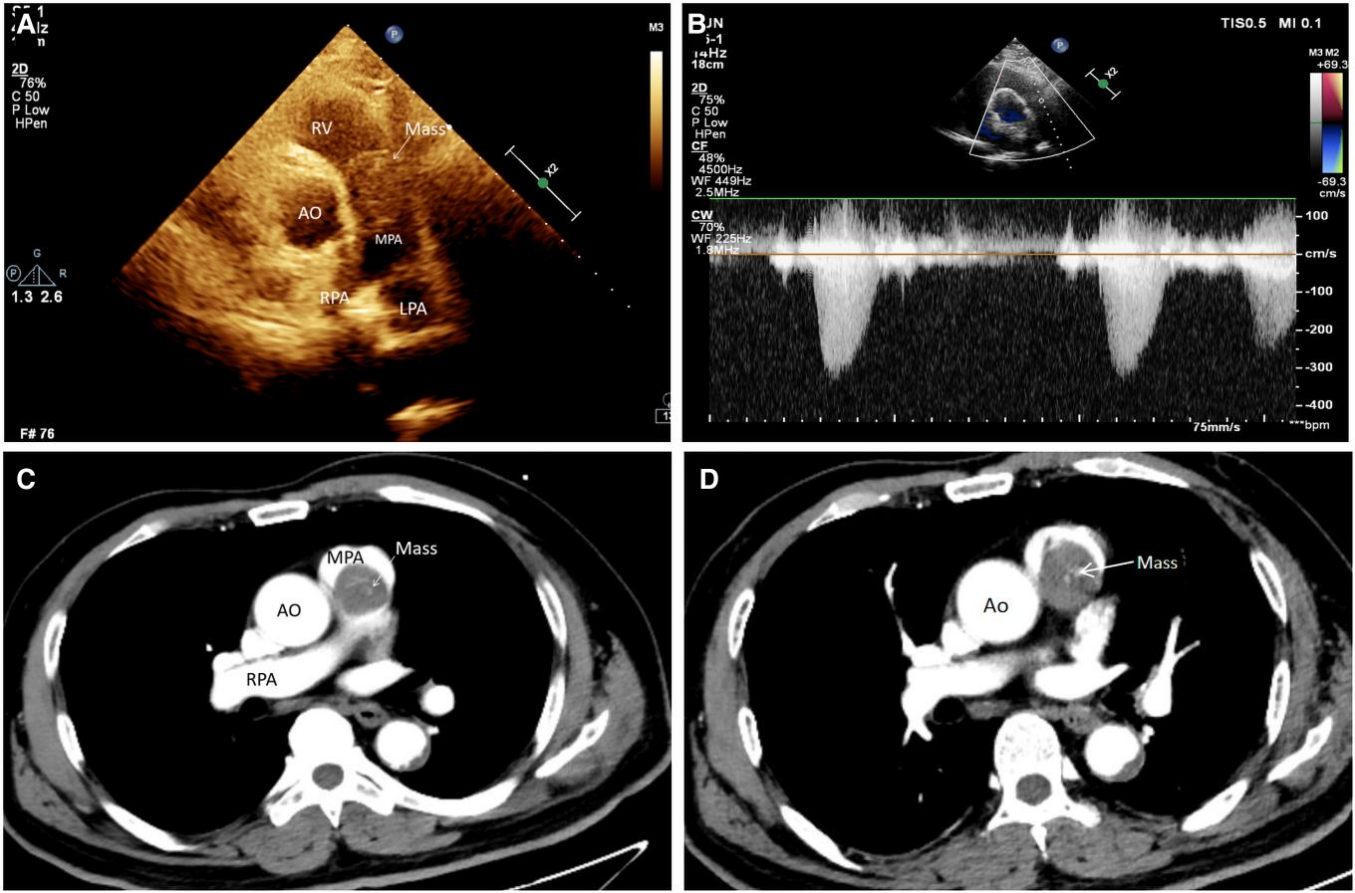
Multimodal imaging. (*A*) Transthoracic echocardiography shows a round-like mass located in the main pulmonary artery trunk. (*B*) Continuous Doppler shows increased blood flow at the mass. (*C* and *D*) Contrast-enhanced computed tomography of the chest shows a mass in the main pulmonary artery at two separate levels. Ao, aorta; RV, right ventricle; MPA, main pulmonary artery; LPA, left pulmonary artery; RPA, right pulmonary artery.
